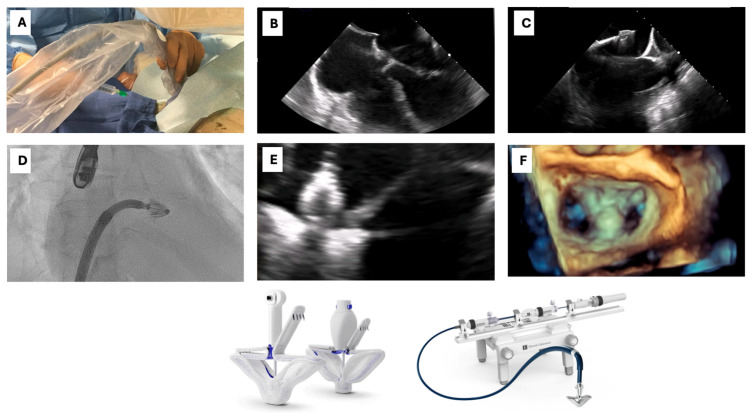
Key procedural steps of transcatheter edge-to-edge repair using the PASCAL system (Edwards Lifesciences). (A) Ultrasound-guided femoral venous puncture using a micropuncture set. (B) Tenting of the interatrial septum during transseptal puncture under transesophageal echocardiography (TEE) guidance (bicaval view), performed using a Mullins transseptal sheath and a Brockenbrough needle. (C) Ultrasound-guided advancement of the PASCAL delivery system (Edwards Lifesciences) into the left atrium (D) Fluoroscopic view showing the PASCAL implant positioned in the left atrium after successful transseptal access. (E) Grasping of the mitral valve leaflets using the PASCAL device under TEE guidance (mid-esophageal commissural view, ~60°), with the paddles and clasps visible. (F) Three-dimensional en face view from the left atrium (LA) showing the mitral valve with the PASCAL device implanted, demonstrating effective leaflet approximation and reduction of mitral regurgitation.
